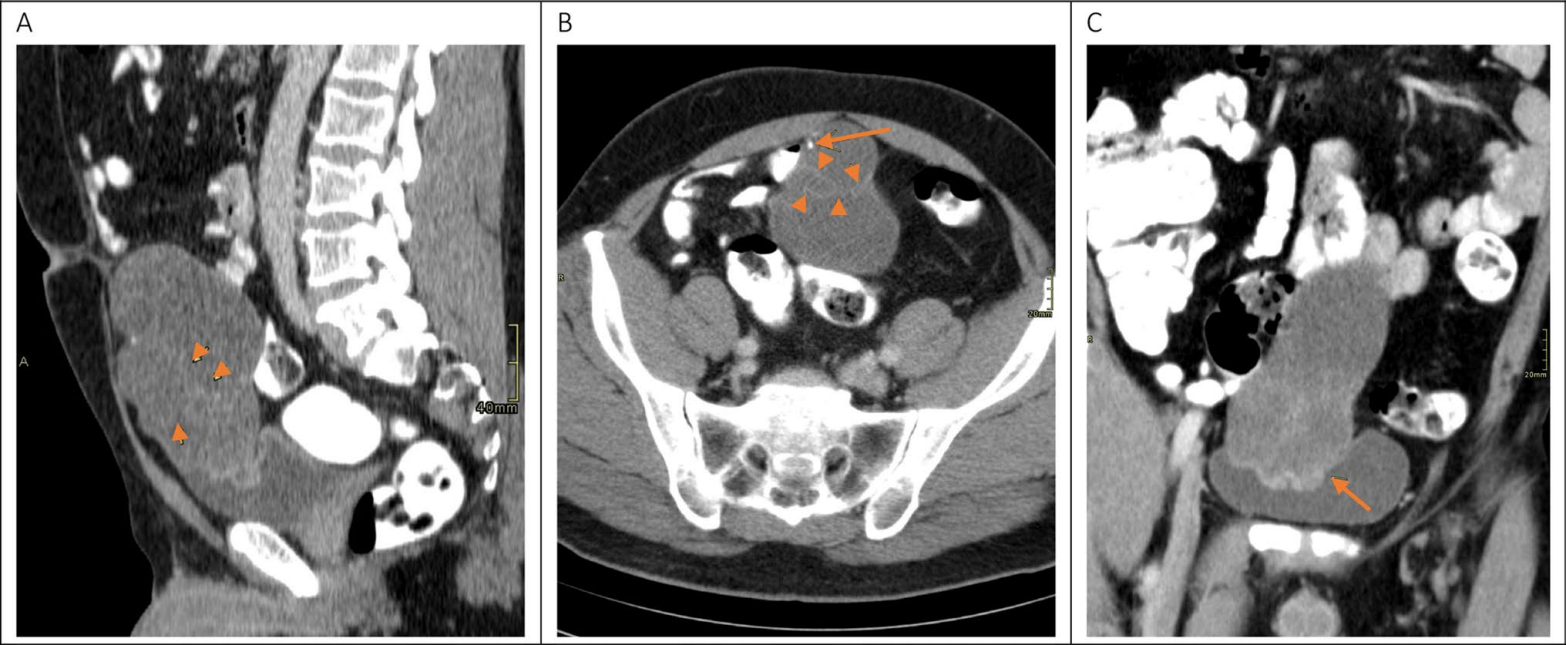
A CT reconstruction in the sagittal plane. A large hypodense lesion is demonstrated in the hypogastrium. This tumor arises from the anterior bladder dome to the level of the umbilicus. The middle umbilical ligament cannot be delineated from the lesion. Some tiny reticular septations are seen in the lesion (arrows). B CT reconstruction in the axial plane. A punctate calcification (arrow) is demonstrated in the periphery of the tumor. Hair thin contrast enhancing septations are crossing the tumor mass. C CT reconstruction in the coronal plane. The base of the tumor cannot be delineated from the muscular layer of the urinary bladder (arrow)
